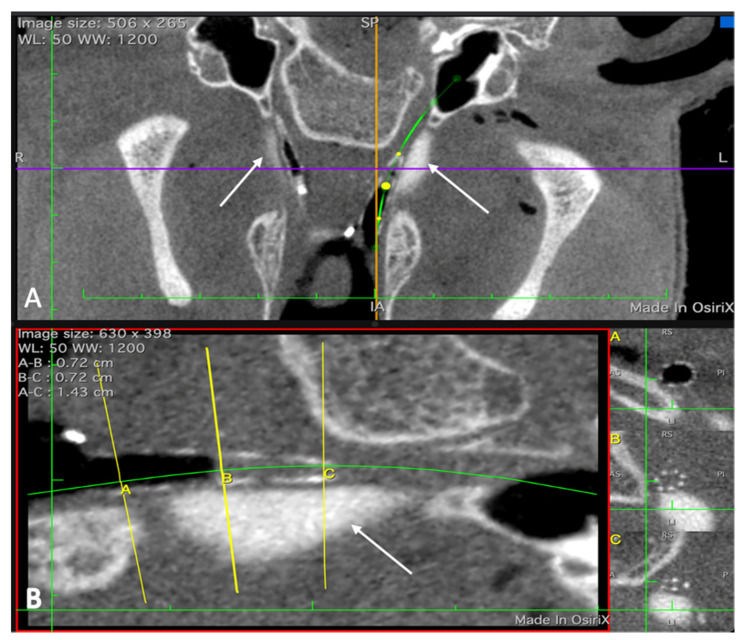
CBCT scan of a head with stent prototypes inserted into the ETs. (A): Both depots (cases 1 (left ET) and 2 (right ET)) are visible and marked by white arrows. Next to the depots, air filled stents can be seen. The green line indicates the course of the left ET. (B): Enlargement of one stented ET with the depot. The yellow lines (A; B; C) mark the position of the respective cross sections depicted on the right side.
